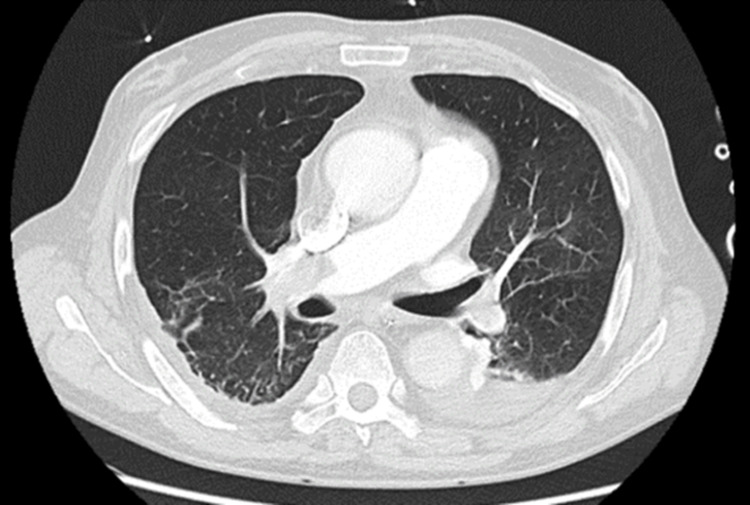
Massive PE on angio-CT. PE: pulmonary embolism.
